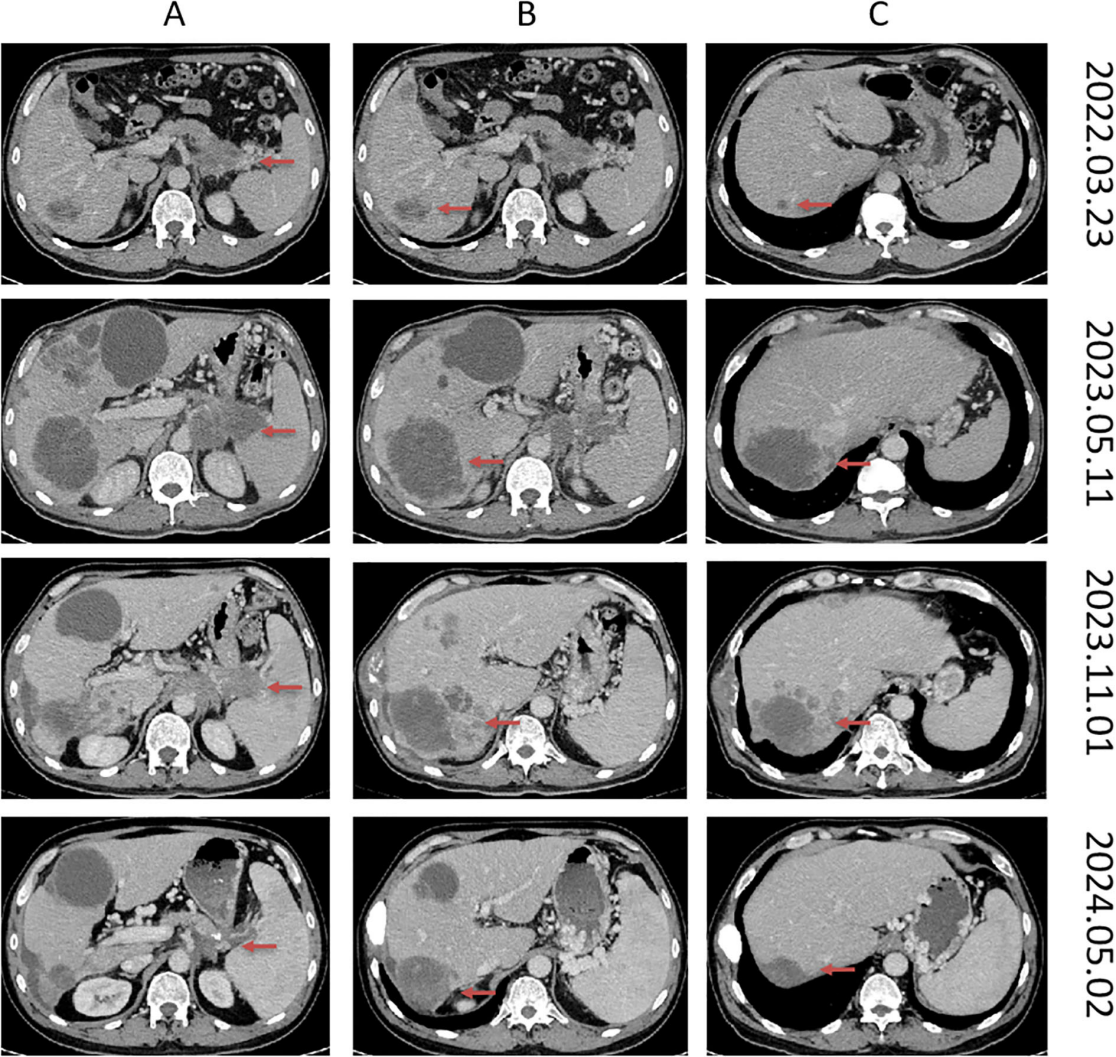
Typical CT images of pancreatic tail cancer and liver metastases before and after treatment at various stages. The red arrow points to the tumor. March 23, 2022 imaging revealed baseline pancreatic cancer lesions along with two liver lesions. May 11, 2023 imaging showed disease progression (PD), which occurred after completion of 13 cycles of FOLFIRINOX chemotherapy. During the treatment course, the patient received maintenance therapy with S-1 for a period, and progression was documented following the final FOLFIRINOX treatment. The November 1, 2023 scan was obtained prior to initiation of liposomal irinotecan plus fluorouracil therapy. The May 2, 2024 scan was obtained after six months of treatment with liposomal irinotecan plus fluorouracil. (A) demonstrates changes in the primary pancreatic lesion before and after treatment. (B, C) represent changes in liver metastatic lesions before and after treatment.
